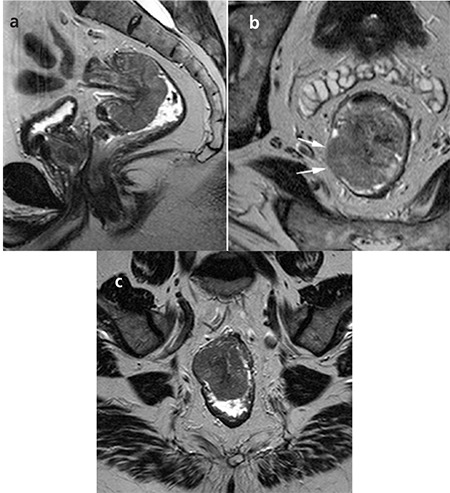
68-year-old man with pT2N1 rectal cancer. Two observersstaged the tumor as T2N0 in the preoperative MR imaging.
